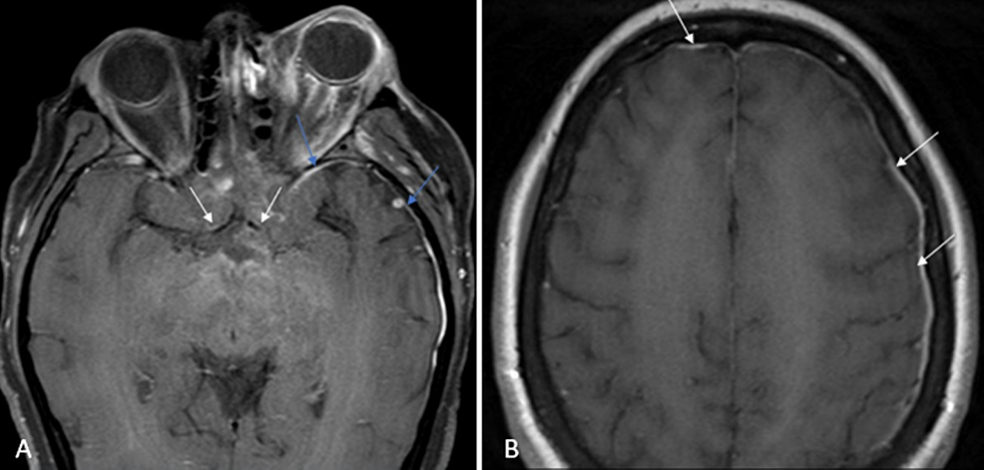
Dural and vascular involvement (A) Axial T1FS TSE post-contrast images demonstrating abnormal perivascular enhancement of the bilateral A1 segments of the anterior cerebral arteries (white arrows) with dural thickening and enhancement (blue arrows); (B) Axial T1 TSE and Axial T1FS TSE post-contrast images demonstrating dural thickening and enhancement. T1FS TSE is T1-weighted fat-suppressed turbo spin echo; T1 TSE: T1 turbo spin echo
